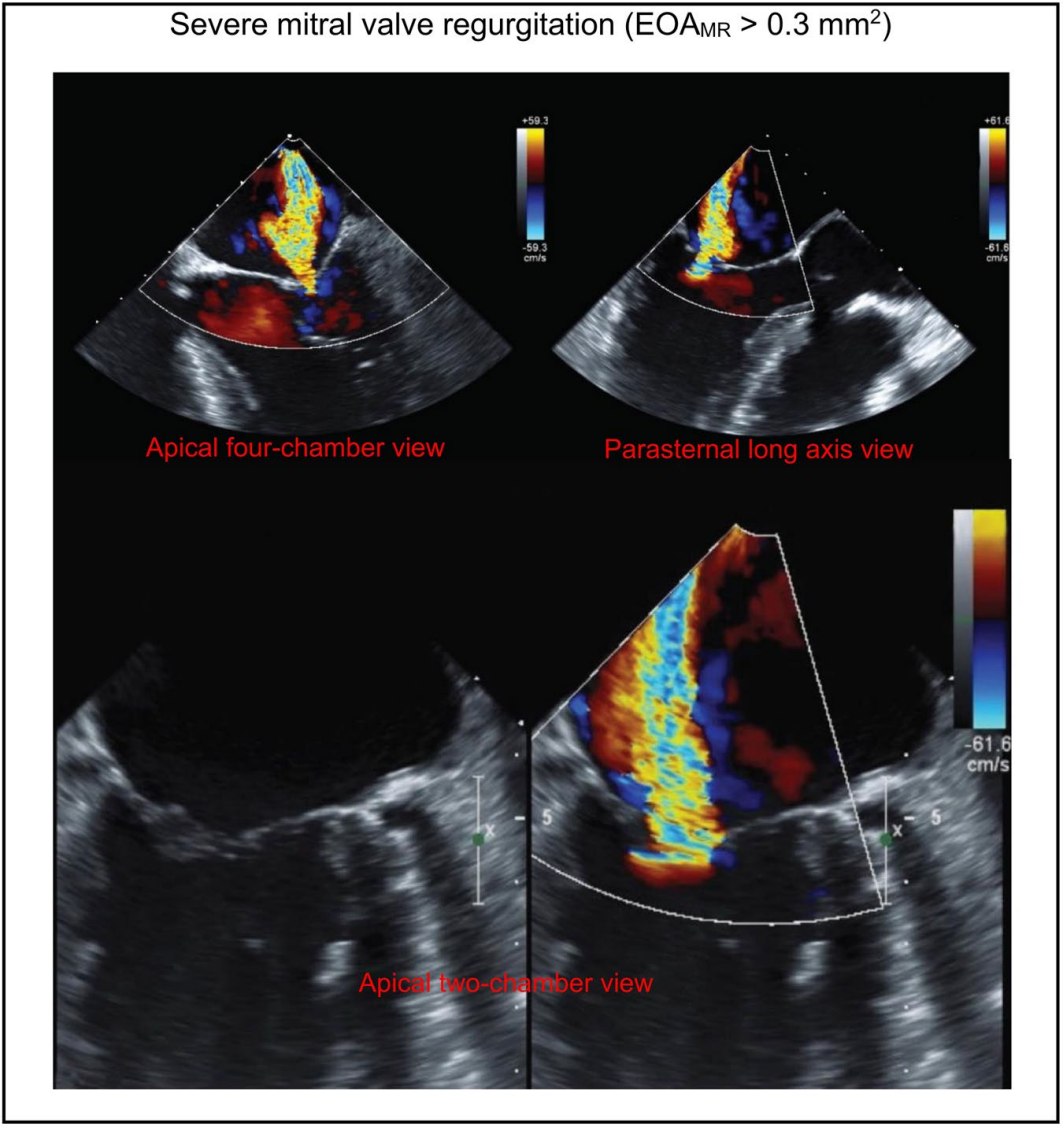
Doppler echocardiography investigation for mitral valve regurgitation. To evaluate mitral valve regurgitation severity, mitral valve color Doppler images are used in apical four-chamber view (top left), parasternal long axis view (top right), and apical two-chamber view (bottom). The three images used are of the same patient, and each demonstrates sever mitral valve regurgitation. This figure is an example of severe mitral valve regurgitation in a patient with AS who received TAVR  EOA_MR_ > 0.3 mm^2^).
